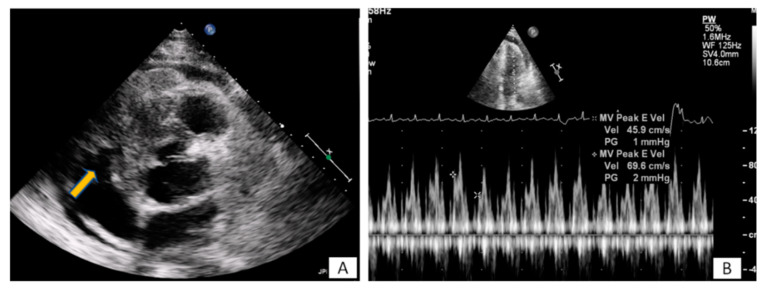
(A) A 65-year-old patient with fever and dyspnea for 15 days. Transthoracic echo (modified short axis view) demonstrated an amorphous mass (arrow) infiltrating the tricuspid annulus, right ventricular free wall and pericardium, causing a moderate in size pericardial effusion. (B) The pericardial fluid led to tamponade (B). Biopsy revealed a lymphoma.
